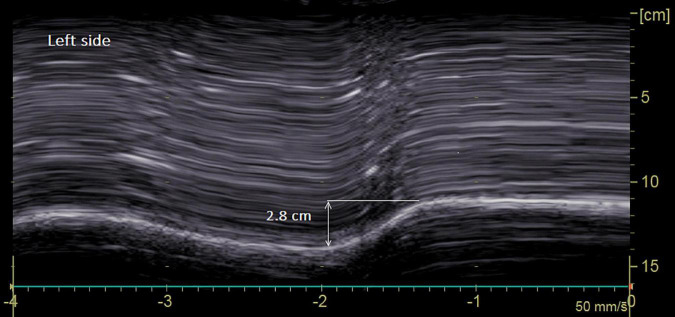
Diaphragmatic motion recorded by M-mode ultrasonography in a man suffering from left hemidiaphragm dysfunction. *On the left side*: marked decrease in hemidiaphragm excursion during deep breathing (2.8 cm for a lower limit of normal = 4.2 cm).
